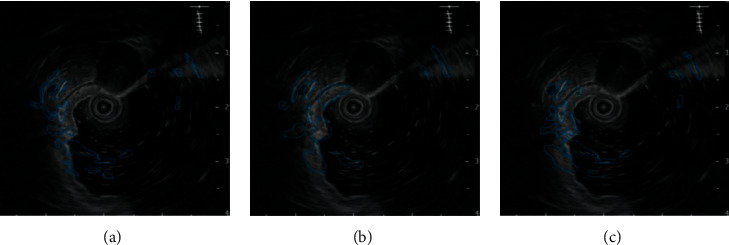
Upper gastrointestinal ultrasonography. (a) Doctor manually identifies early cancer lesions. (b) CNN model identifies early cancer lesions. (c) Cascade RCNN identifies early cancer lesions.
